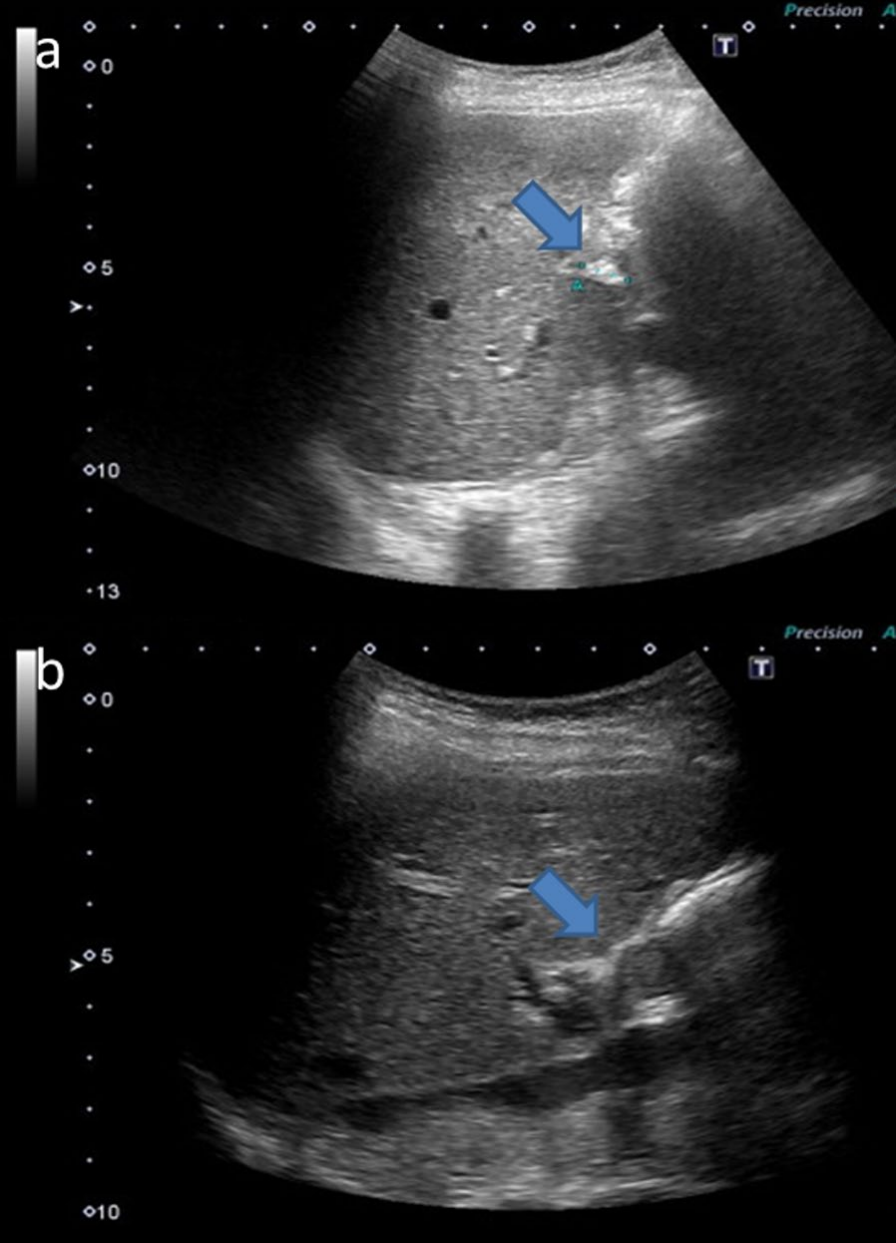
Abdominal ultrasound (AUS) findings. a AUS performed 6 months after the colon surgery. A Highly echoic structure is visible in the cystic remnant gallbladder (arrow). b AUS performed 9 months after the colon surgery. A 13-mm isoechoic solid lesion is visible in the remnant gallbladder (arrow)
